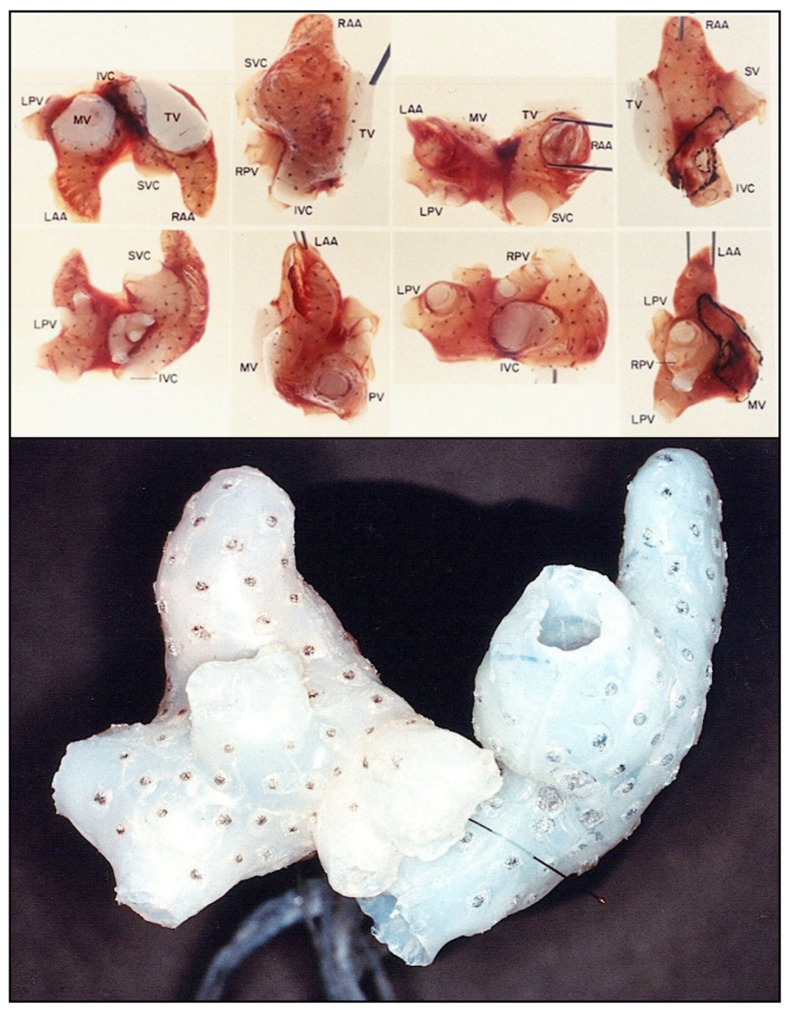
Three-dimensional electrode arrays used for the experimental mapping (upper panel) and clinical mapping (lower panel) of atrial fibrillation in the mid-1980s. The individual bipolar electrodes were newly designed “target electrodes” that had the anode as the central point and the cathode in a circular pole surrounding the central anode. This design eliminated potential artifact cause by differences in the direction of wavefront propagation. The three-dimensional electrical data was then superimposed on the three-dimensional anatomic reconstruction of the same atria using gated MRI scans (Figure 6). The experimental electrode arrays contained 256 individual bipolar electrodes and the clinical electrode arrays contained 156 individual bipolar electrodes. Both electrode arrays were designed and created by Dr. John P. Boineau and Dr. Richard B. Schuessler.
